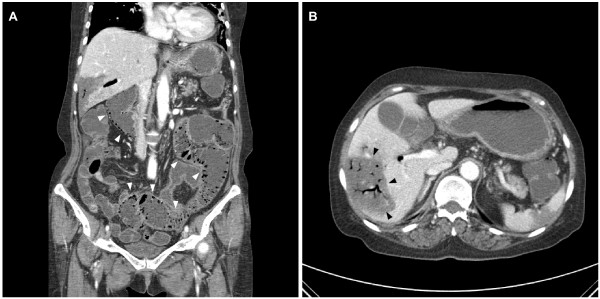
Computed tomography (CT) image of the abdomen and pelvis. The CT scan shows extensive bubble-like pneumatosis intestinalis along the entire colon (A, white arrows), branching lucencies indicating hepatic portal venous gas, and a low-attenuation lesion in the right lobe of the liver, suggestive of infarction of the liver (B, black arrows).
